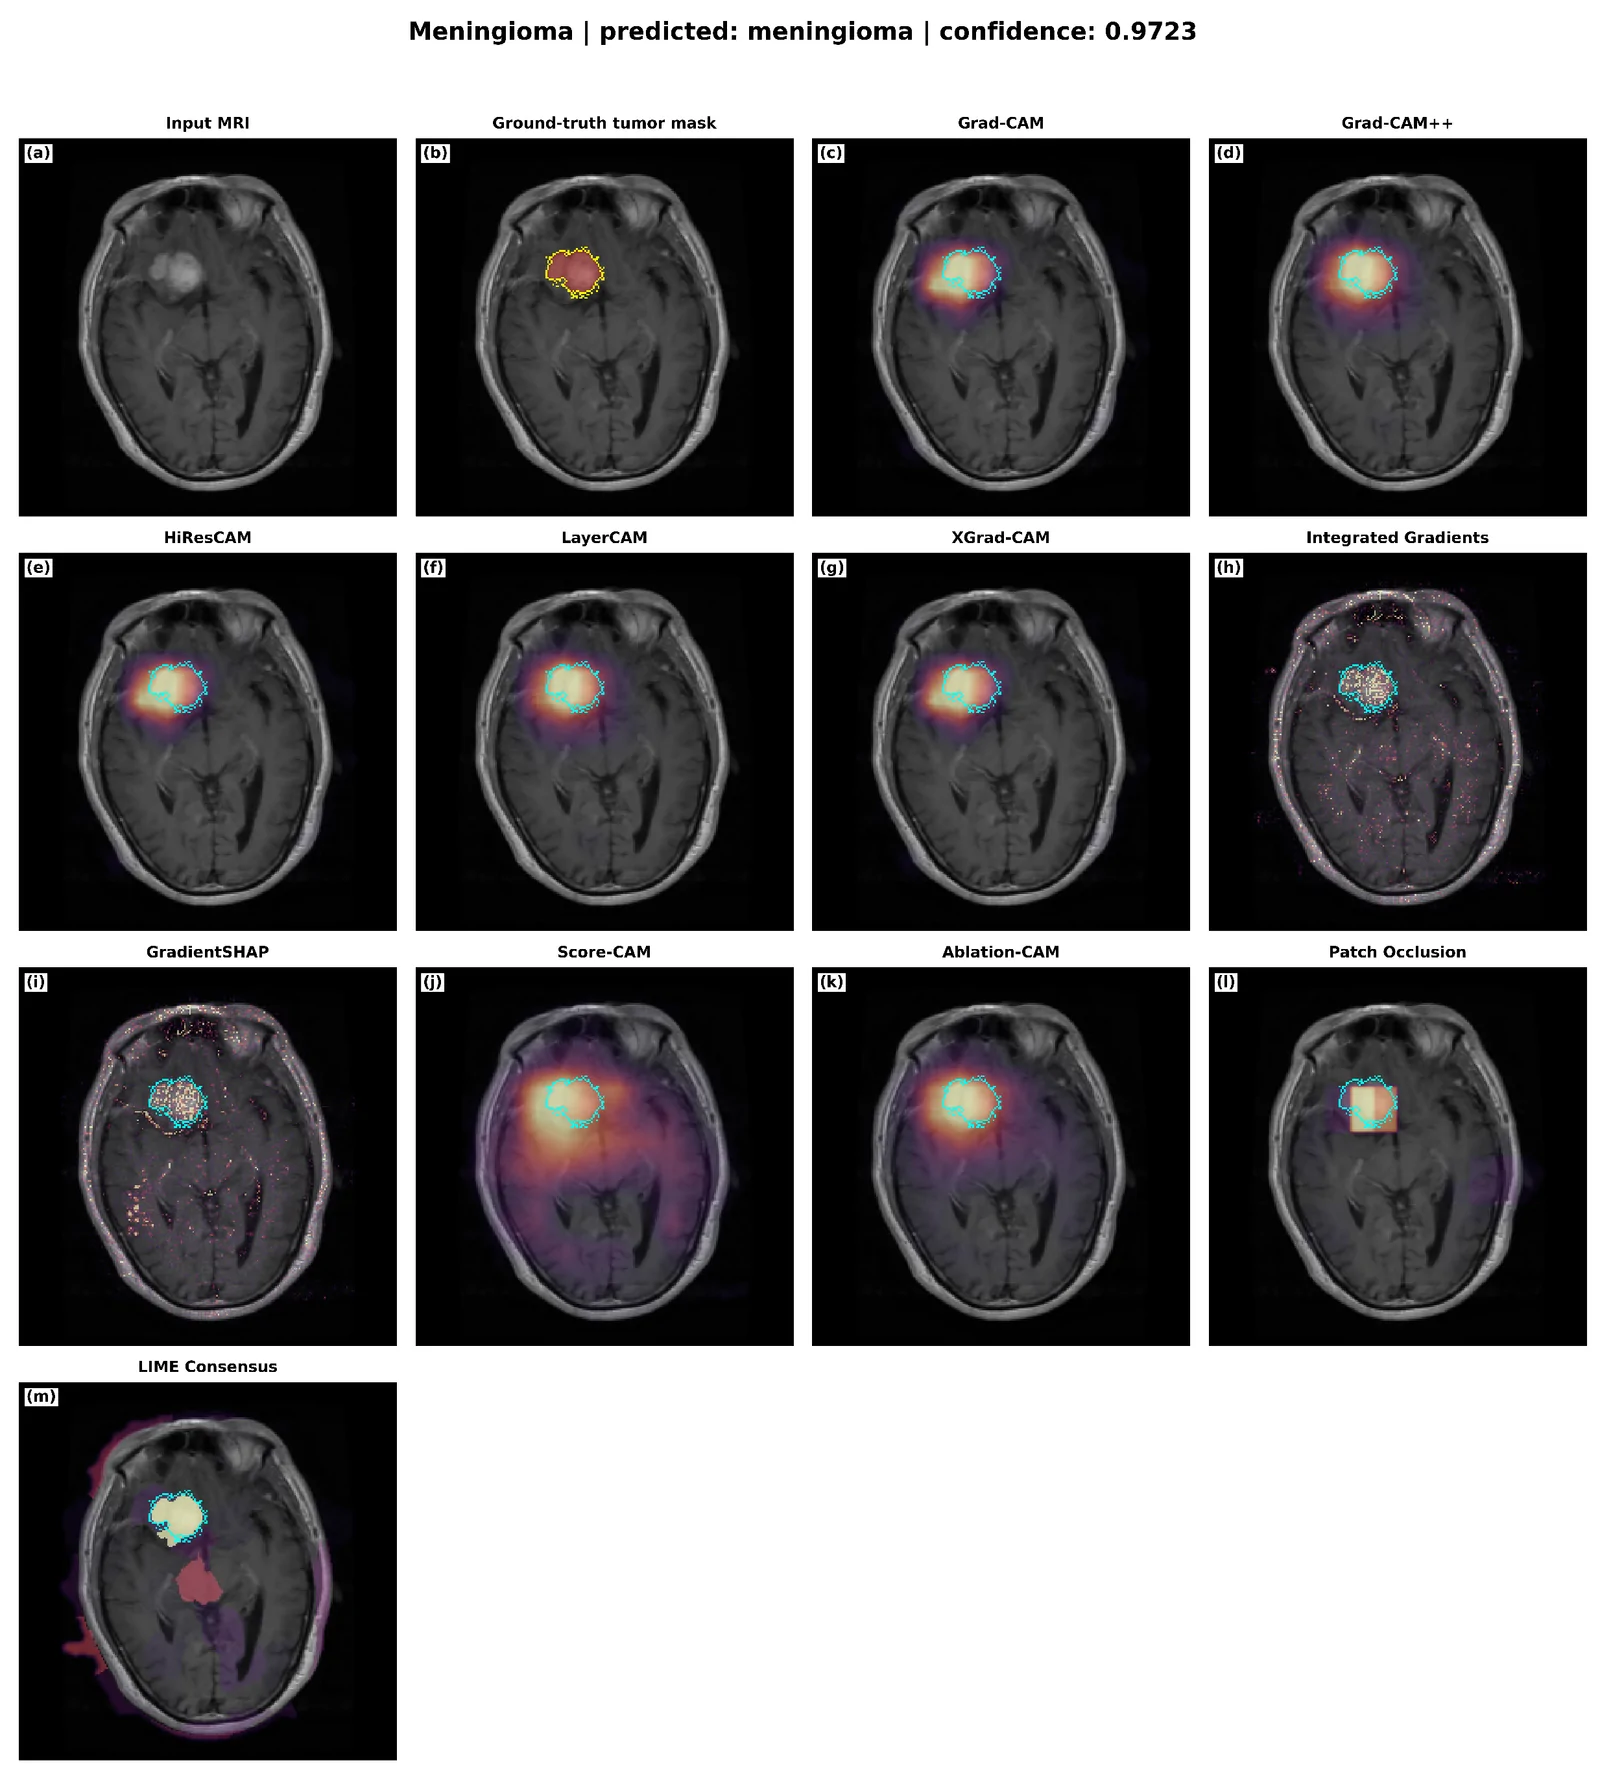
Visualization of all evaluated XAI methods for a meningioma MRI image.
